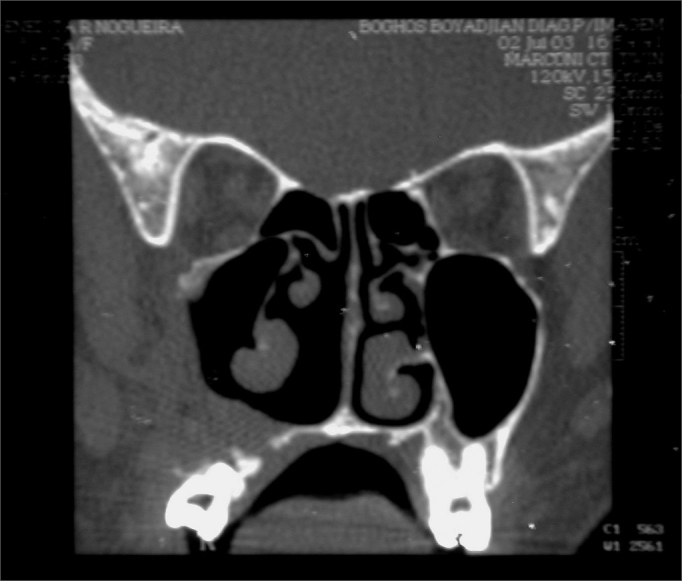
CT scan - coronal view of a GCCG post operative (case 2).
